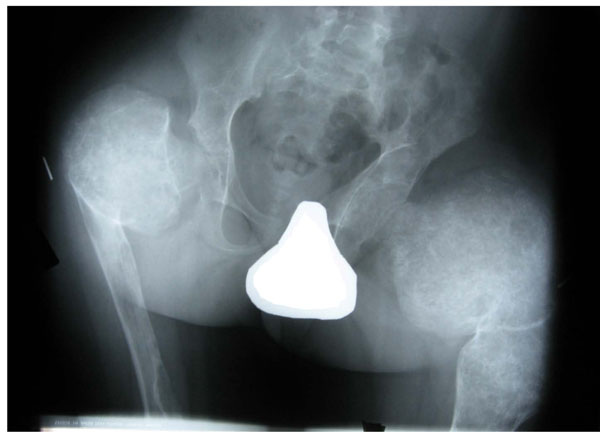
Fibrous dysplasia of the proximal femur. The radiograph demonstrates severe femoral involvement with deformity, and a typical ground glass appearance in both proximal femora.
